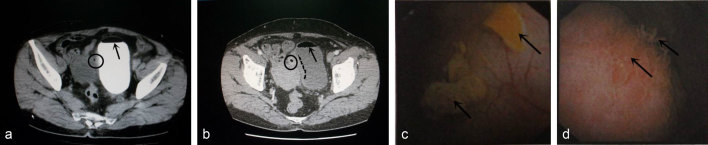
(a) CT scan shows gas (arrowhead) and contrast medium within the bladder, and (b) thickening on the right aspect of the bladder wall (dotted line) with a localized mass (~2.5 × 1.7 cm) and small gas bubbles(circle) were visible. The CT value was 37 Hounsfield units and the enhanced CT value was 72 Hounsfield units. Cystoscopy (c,d)shows bullous oedema and mucosal papillomatous hyperplasia(arrow)
